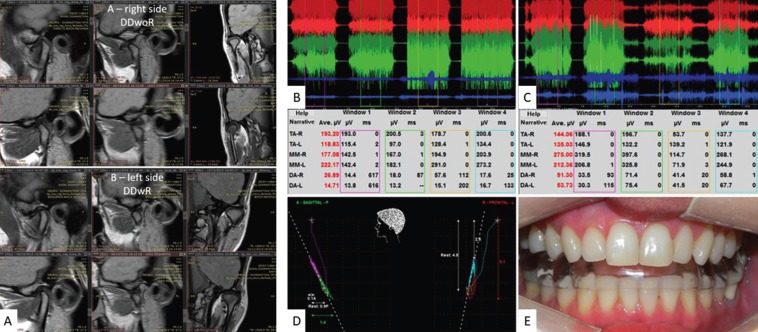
A) MRI in PD right side. Pretreatment (upper) in sagittal closed mouth with anterior disc displacement; open mouth without disc recapture and coronal with decreased joint space. Post-treatment (lower) in open and closed mouth with recapture of disc and increased joint space; coronal in the closed mouth showing joint decompression and repositioning of the articular disc. Left side MRI. Pretreatment (upper) in sagittal closed mouth with anterior disc displacement; open mouth with disc recapture and coronal with decreased joint space. Post-treatment (lower) in sagittal closed and open mouth with good disc placement and increased joint space; coronal showing joint decompression and repositioning of the articular disc in closed mouth. B) EMG/EGN. Measurement parameters before and during decompression treatment. Joint decompression test before treatment (B) and post-treatment (C) with cotton rolls demonstrating maximum occlusion, right side roll occlusion, left side roll occlusion and bilaterally roll occlusion, In (B) with improvement muscle recruitment after increasing the vertical dimension. D) Take of the neurophysiological record from the mandibular three-dimensional / orthopedic positioning resulted from the neuromuscular deprogramming. E) view of occlusion with the use of the intraoral orthotic (IO).
